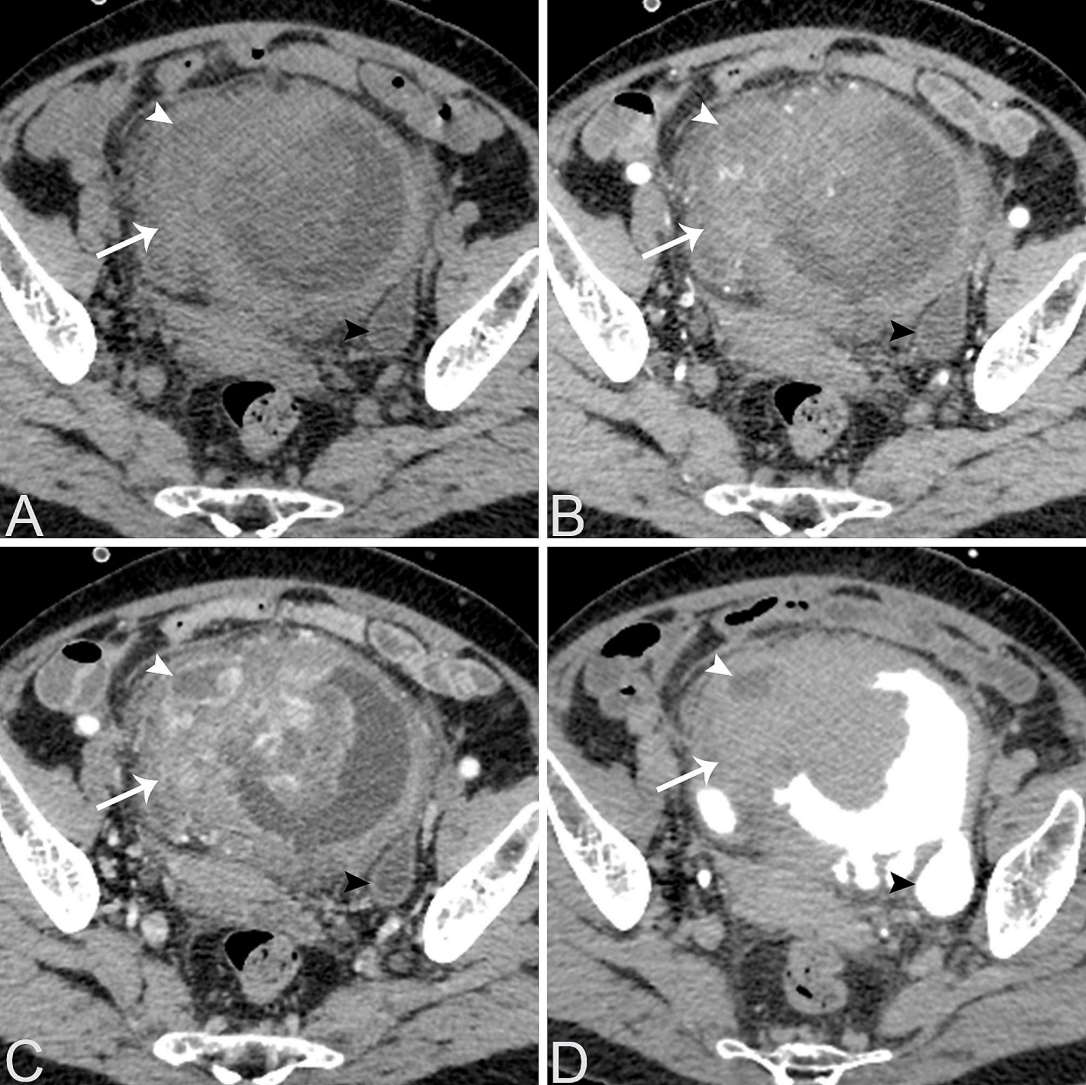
A 69-year-old female patient with bladder SUC involving the right bladder wall diffusely. Axial non-contrast CT (A) shows an ill-defined, lobulated, and slightly hypodense lesion in the diffuse bladder wall, predominantly involving the right wall (arrow). Contrast-enhanced CT images in the corticomedullary phase (B), nephrographic phase (C), and excretory phase (D) reveal heterogeneous and marked enhancement of the mass (arrow) with small focal areas of necrosis (white arrowheads). A local bladder diverticulum was noted in the left posterior wall (black arrowheads)
